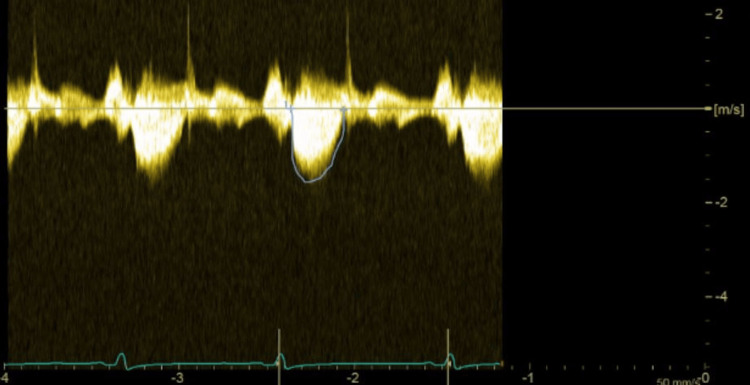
TEE image of continuous wave Doppler with no significant obstruction through the LVOT with the IABP at 1:3 counterpulsation. TEE: transesophageal echocardiography; LVOT: left ventricular outflow tract; IABP: intra-aortic balloon pump
